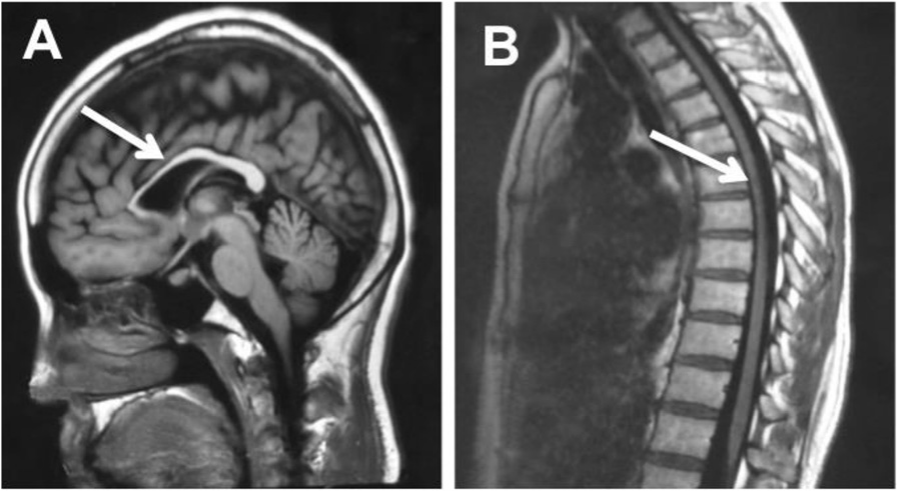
Sagittal brain and thoracic spinal MRI image in index patient (II:3). a: Brain MRI shows thinning of corpus callosum, emphasized in the anterior part with a “beaked” shape (*arrow*). Ther is no obvious periventricular or deep cerebellar white matter lesions and no obvious cerebral or cerebellar atrophy. b: Throracic spinal MRI shows thinning of the thoracic spinal cord with volume loss but no obvious signal abnormalities in the cord
